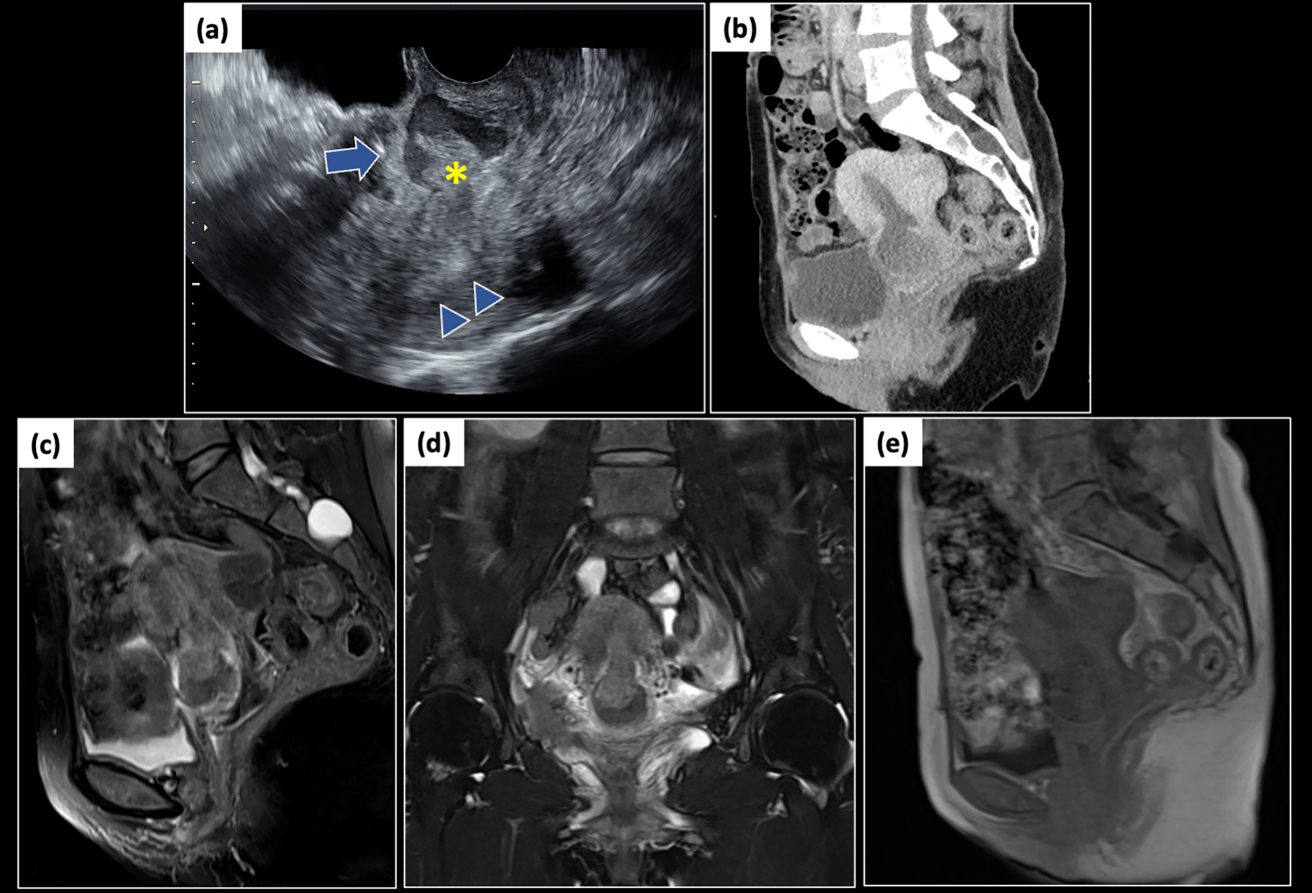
Imaging findings of hematometra within cesarean scar defect. (a) Transvaginal ultrasonography revealed the existence of hematometra within CSD and high‐echoic objects flowing back and forth between the uterine cavity and CSD. The arrow indicates CSD, the triangles indicate the uterine cavity, and the asterisk indicates high‐echoic objects. (b) CT scan revealed no evidence of extravasation surrounding the CSD. (c, d) MRI on T2WI: (c) a sagittal section; (d) a coronal section. CSD was hypointense, and the blood flowing from the uterine cavity into it was hyperintense. (e) MRI on T1WI of sagittal section. Both the CSD and the blood flowing into it were slightly hypointense. CSD, cesarean scar defect; CT, contrast‐enhanced computed tomography; MRI, magnetic resonance imaging; T2WI, T2‐weighted images; T1WI, T1‐weighted images.
